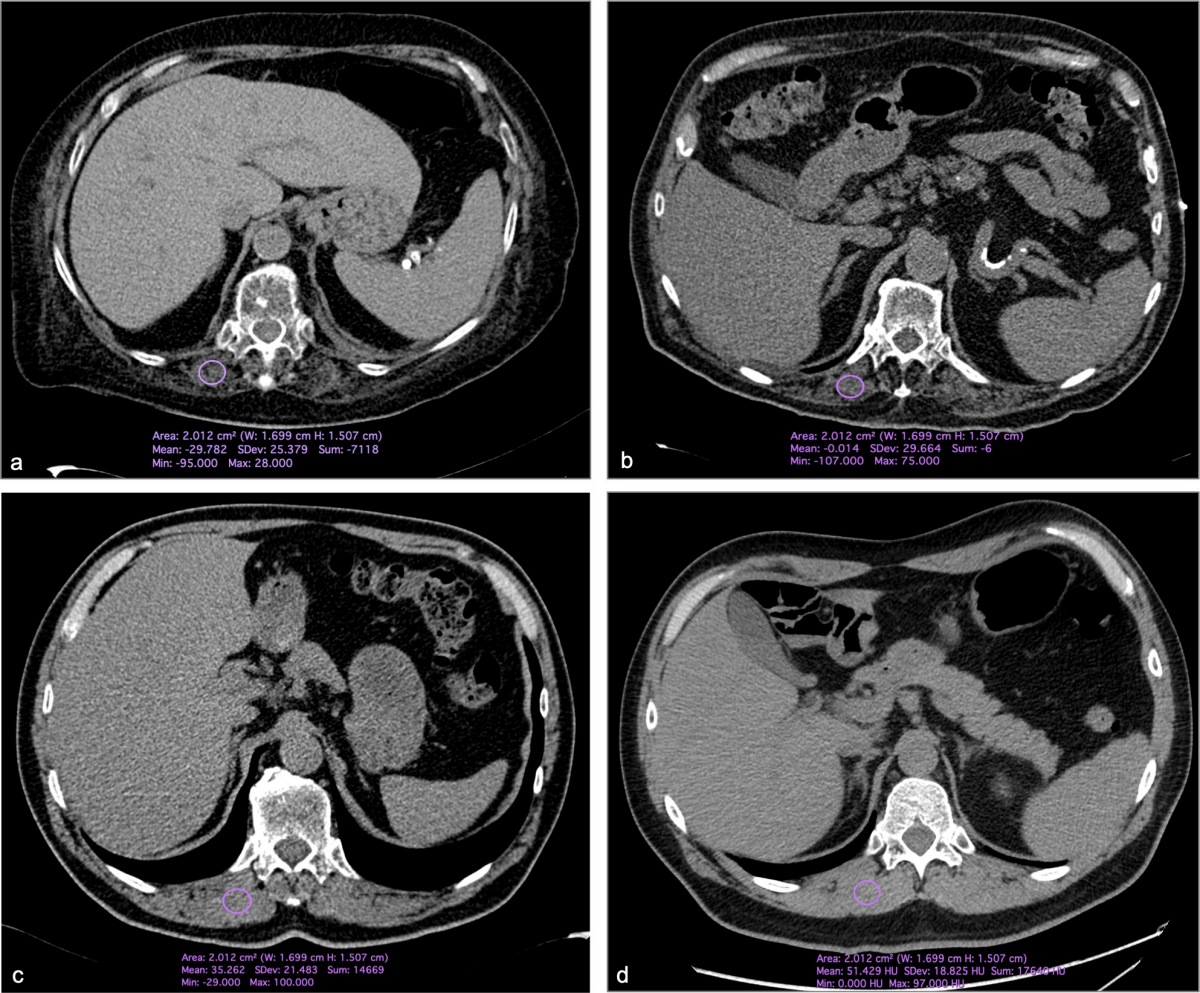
Axial Computed Tomography images of four patients with COVID-19. In particular, in a and b, one 74 years-old female and one 82 years-old male with reduced muscle mass (i.e., < 30 Hounsfield unit) hospitalized in intensive care unit. In c and d, two male patients (54 years old in a and 65 years old in b) hospitalized in COVID-19 wards demonstrating muscle values above the threshold for muscle loss.
